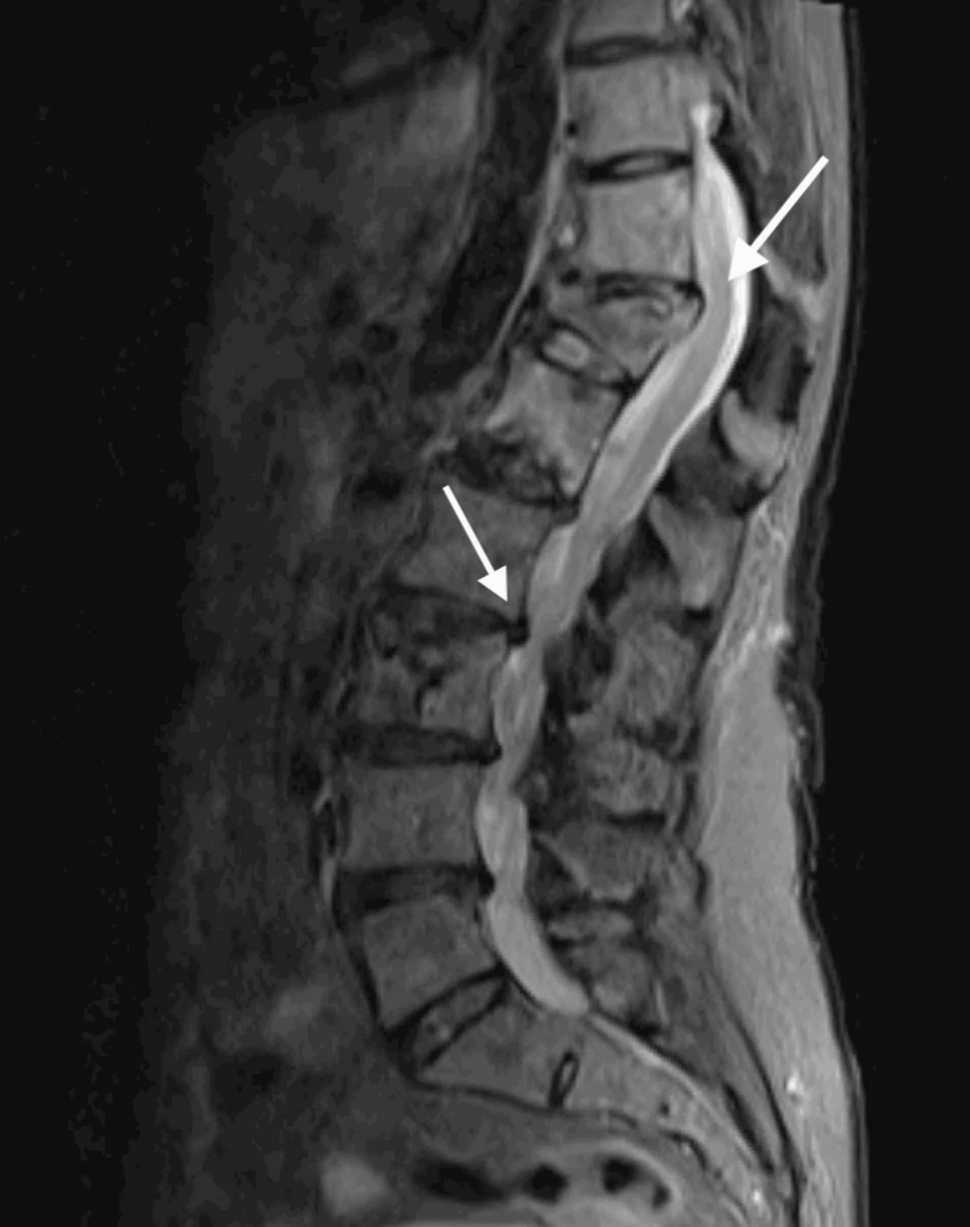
Sagittal T2-weighted spinal MRI showing multilevel degenerative changes without evidence of myelopathy MRI: magnetic resonance imaging
